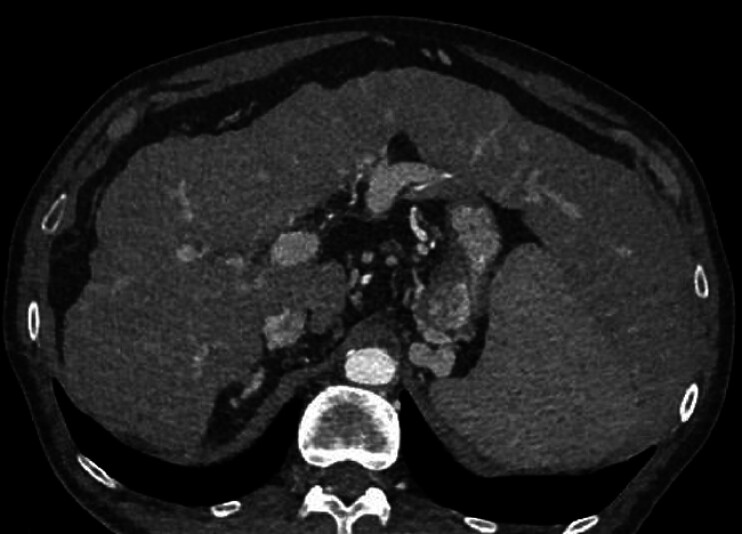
CT angiography showed cirrhosis, splenomegaly, and portal hypertension with gastroesophageal varices and portosystemic collateral vessels.
